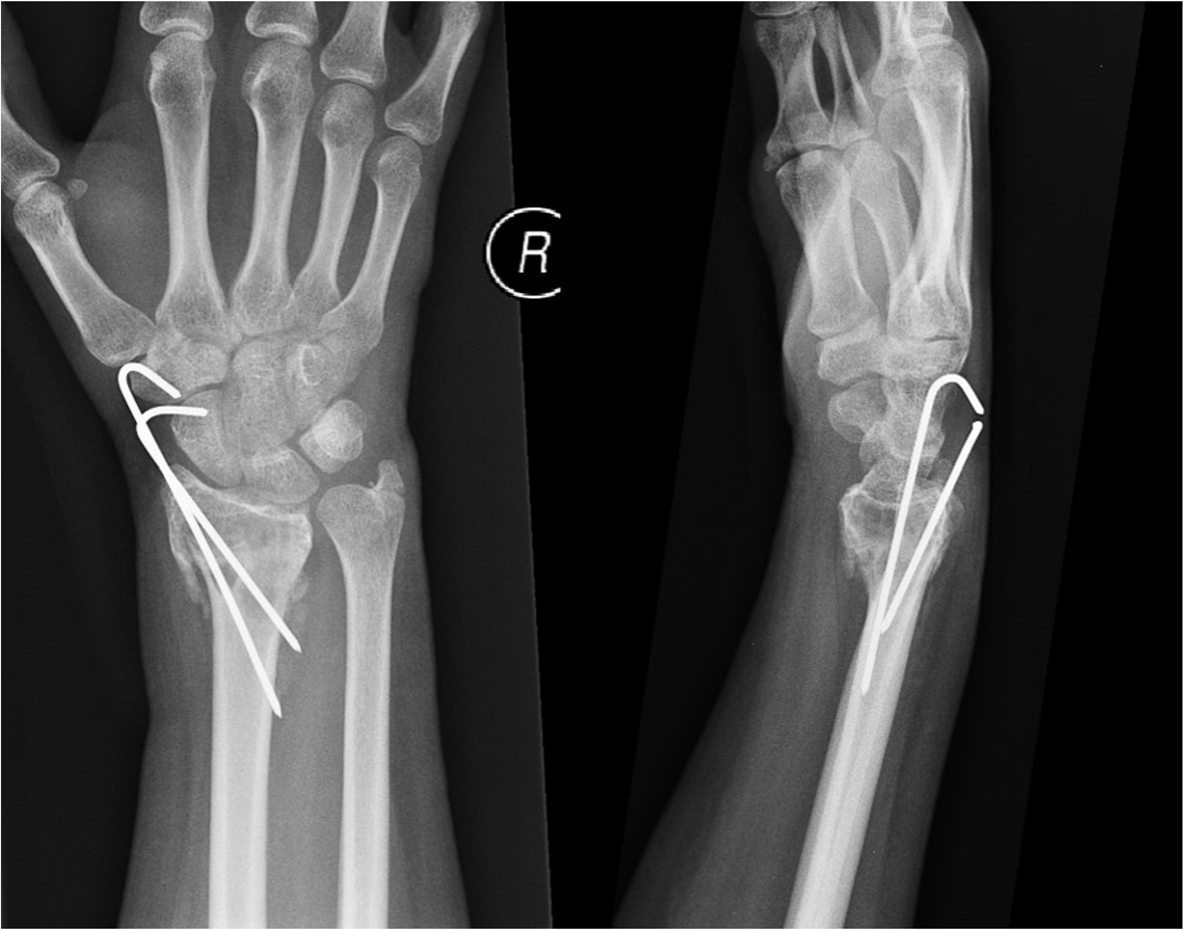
ap and lateral plain radiographs of right wrist 6 weeks after fixation and start of denosumab
